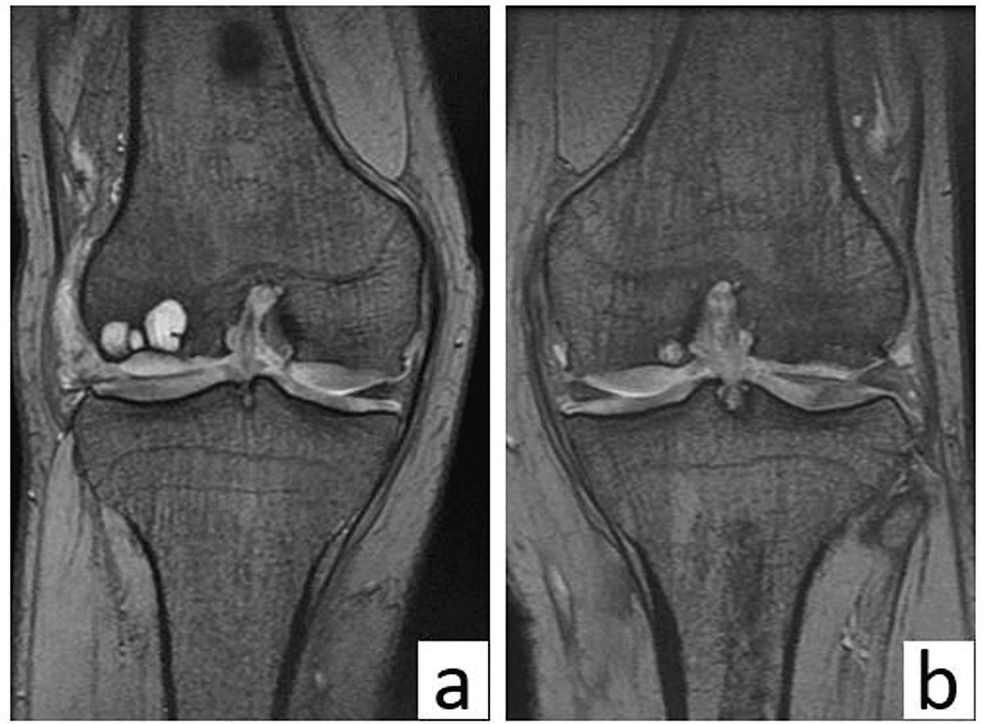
Magnetic resonance imaging of both knees prior to surgery (a) Coronal T2-weighted magnetic resonance image of the right and (b) left knees.
